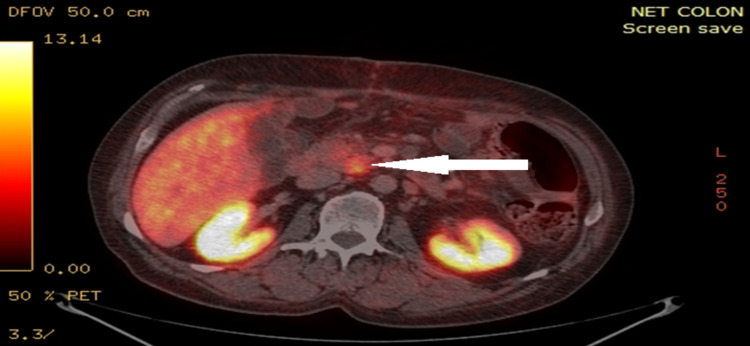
Somatostatin receptor imaging with gadolinium Ga 68–DOTATATE PET/CT at third month postoperatively The image shows high isotope uptake of retroperitoneal lymph node (arrow) suggestive of metastatic disease PET/CT: positron emission tomography/computed tomography
